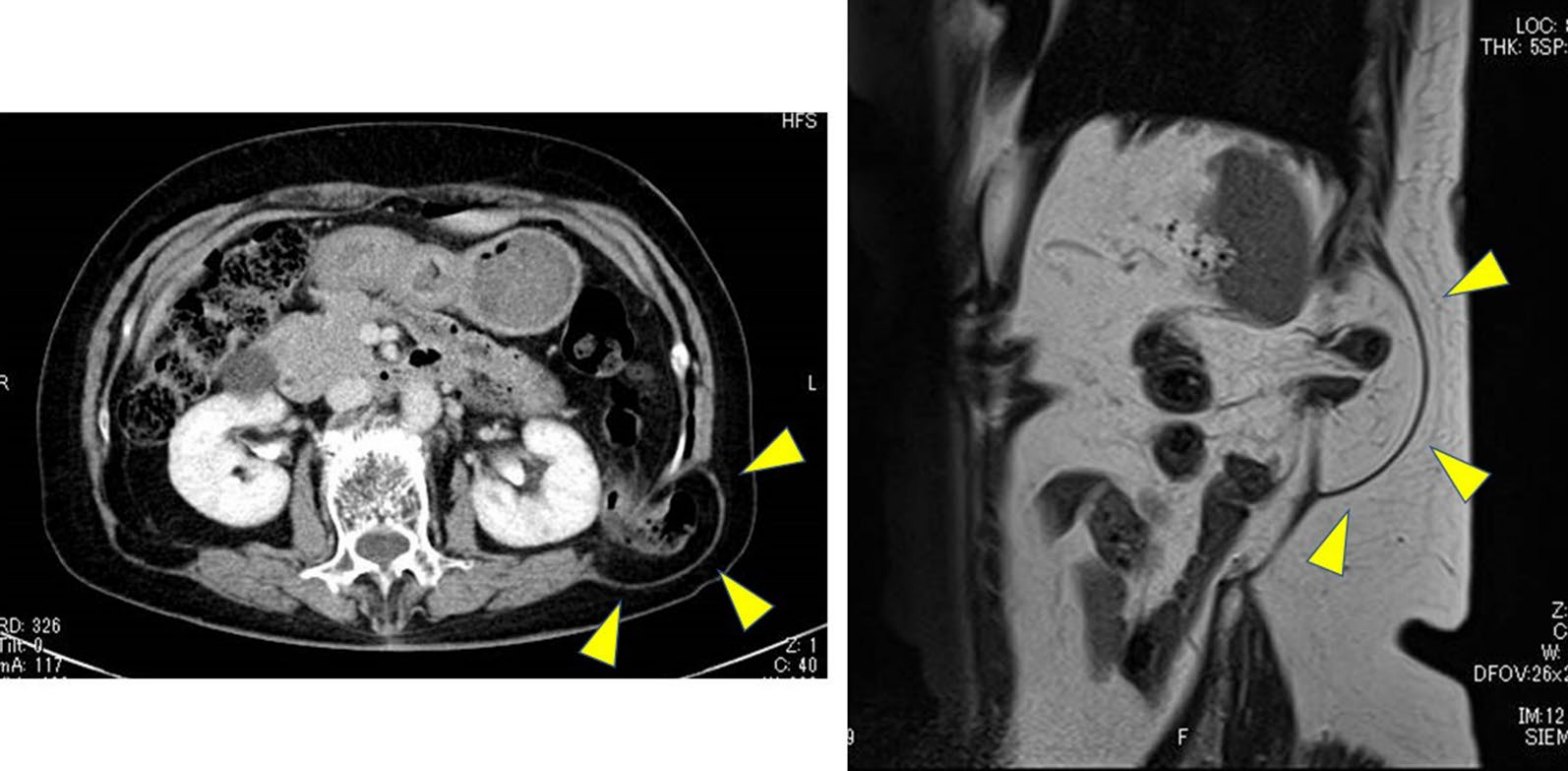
Abdominal CT and MRI. The left: Preoperative computed tomography image. The right: preoperative magnetic resonance image. A fascial defect was in the left lumbar abdominal wall and in which retroperitoneal fatty tissue and the descending colon protruded (arrows)
